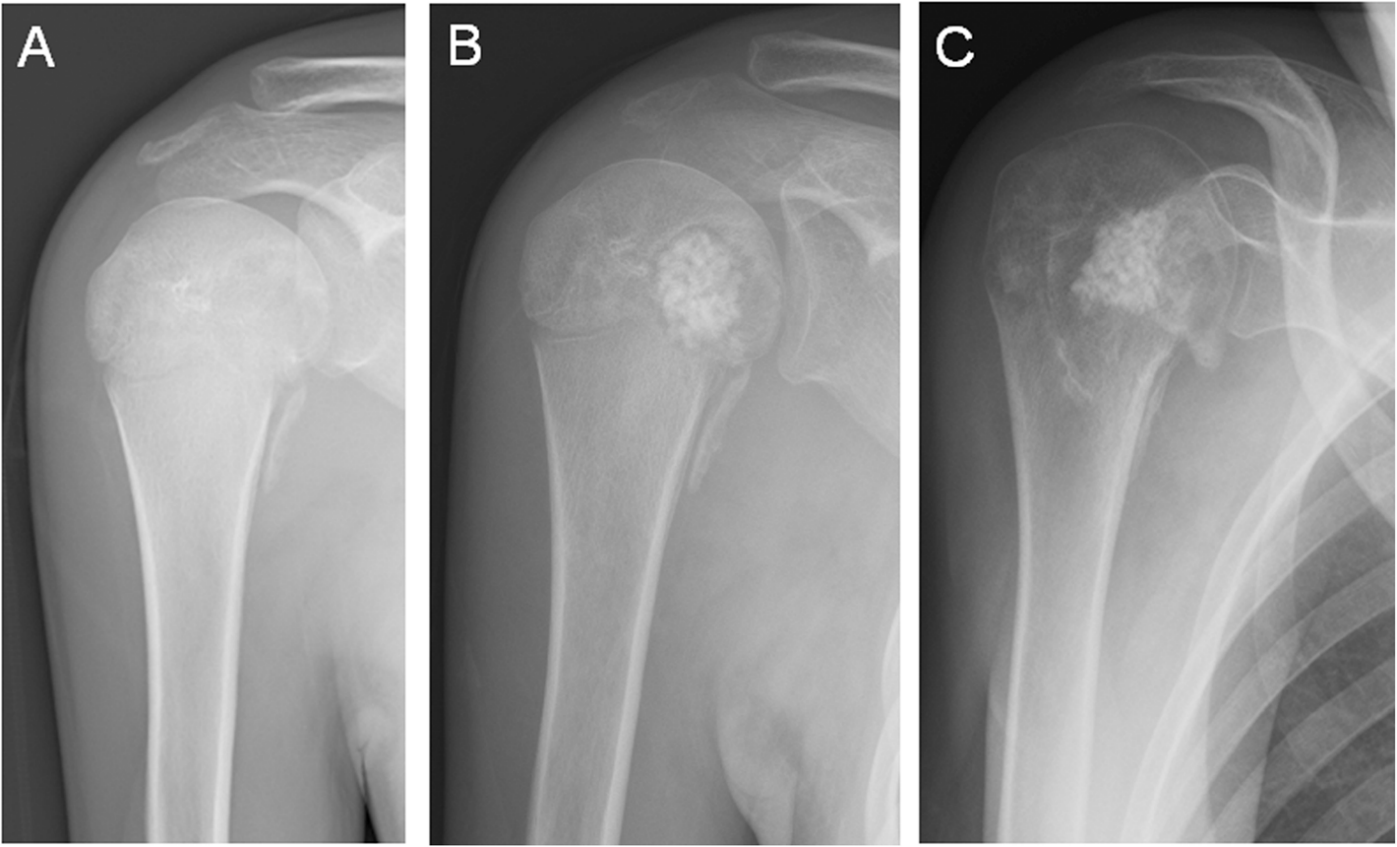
Chondroblastoma arising from the right proximal humerus. a Plain radiography upon referral showing a lytic lesion in the proximal humerus. b One month after surgery. c One and a half year after surgery. This patient had mid shoulder pain
